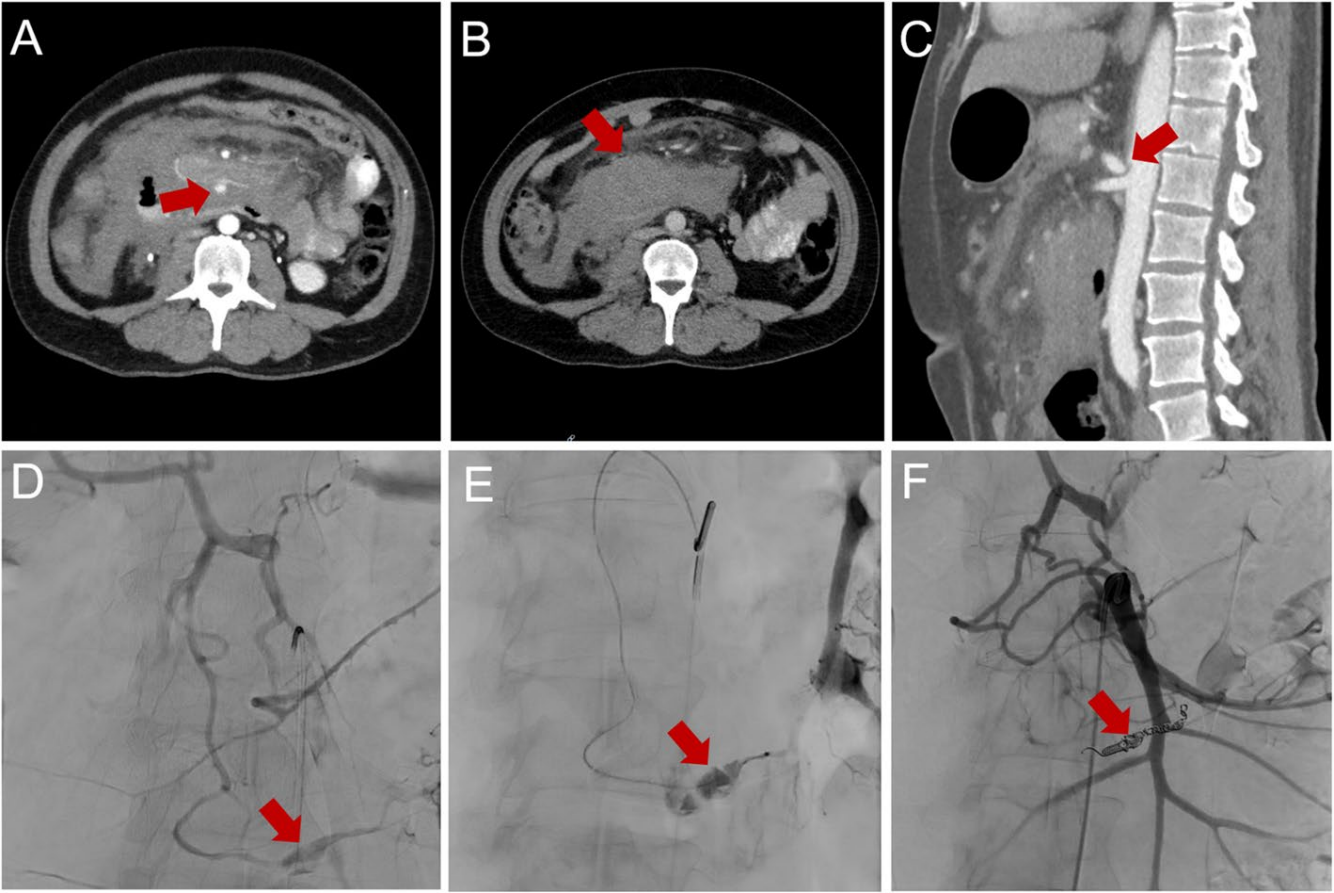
Representative case. MIP CTA and DSA imaging of a 44-year-old male patient with admission to our clinic with upper abdominal pain. A CTA showed high grade CA stenosis and a ruptured 10 mm pseudoaneurysm of the pancreaticoduodenal arcade in a non-critical position (red arrow) with (B) surrounding hematoma (red arrow). D Since probing the CA was extremely difficult at the beginning of the intervention, the initial findings were presented via mesentericography, where the well-known aneurysm was detected as a subtle contrast blush (red arrow). E Successful probing of the CA with a reversed curve catheter and super selective probing of the pancreaticoduodenal arcade. F Front-and-back-door coil-embolization of the pancreaticoduodenal arcade aneurysm was successful
